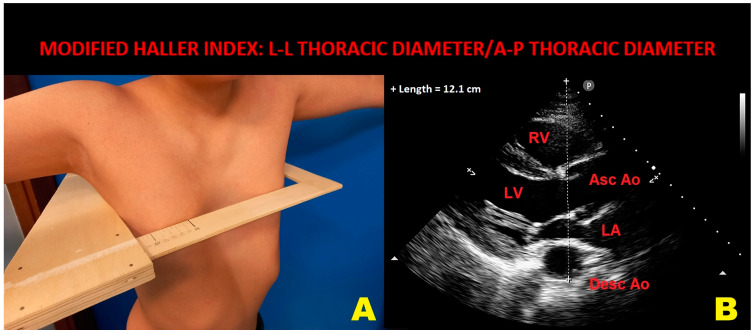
An example of MHI assessment in a PE individual. Panel (A): L-L thoracic diameter, measured with the individual in the standing position and with open arms by using a rigid ruler in centimeters coupled to a level (the measuring device), placed at the distal third of the sternum, at the point of maximum depression of the sternum. Panel (B): A-P thoracic diameter, obtained with the individual in the left-lateral decubitus position during conventional transthoracic echocardiography by placing a 2.5 mHz transducer near the sternum in the left third or fourth intercostal space, to obtain a parasternal long-axis view, and measuring the distance between the true apex of the sector and the anterior surface of the vertebral body. The vertebral body is identified by using, as a reference point, the posterior wall of the descending thoracic aorta, visualized behind the left atrium. Ao, aorta; A-P, antero-posterior; Asc, ascending; Desc, descending; LA; left atrium; L-L, latero-lateral; LV, left ventricle; MHI, modified Haller index; PE, pectus excavatum; RV, right ventricle.
